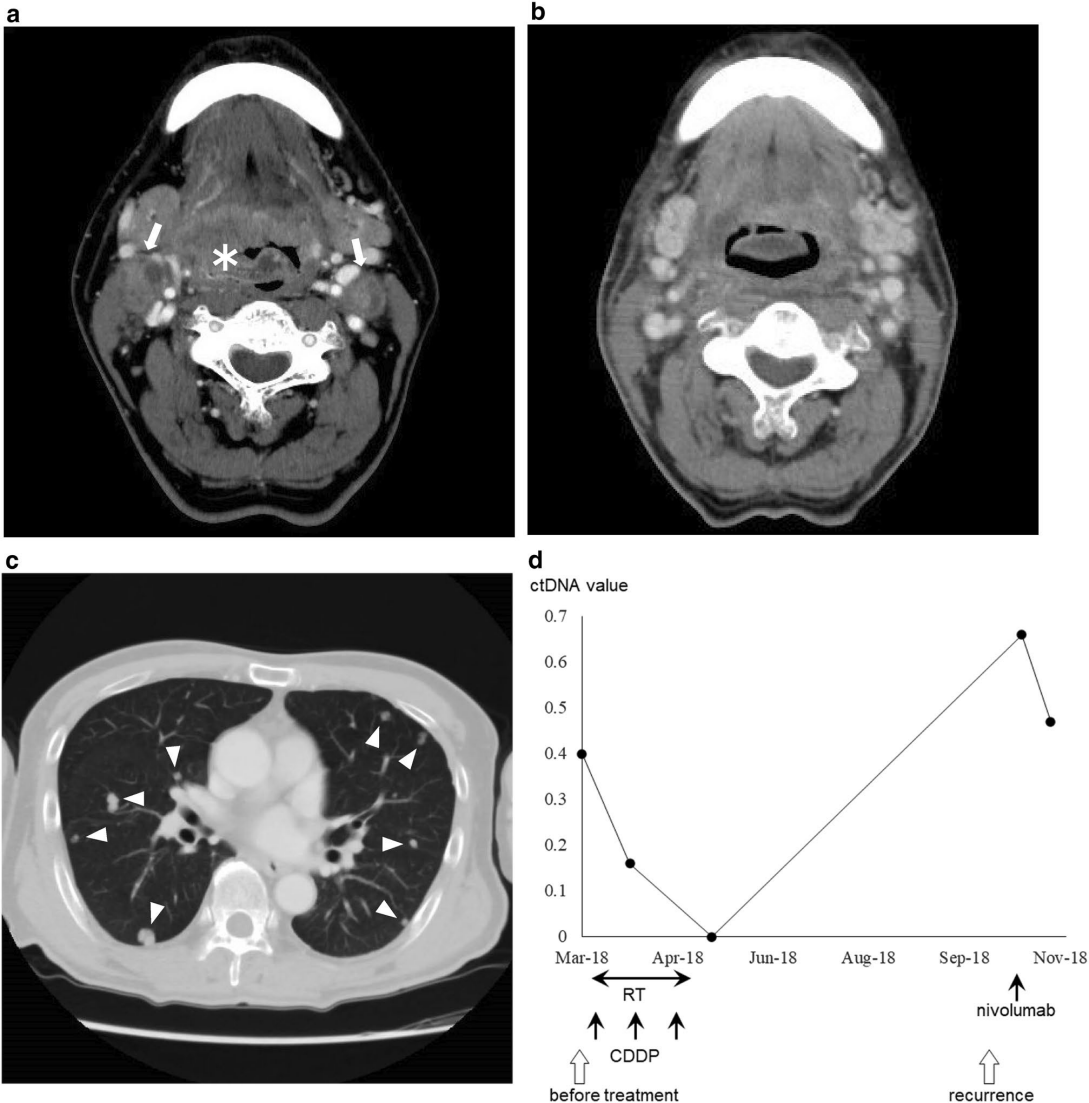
(a) Pretreatment computed tomography (CT) image shows the tumor (asterisk) extending from the right lateral wall to the anterior wall of the oropharynx and cervical node metastasis (arrows). (b) CT image after initial treatment shows complete response. (c) CT image shows multiple lung metastases (triangles). (d) The pretreatment circulating tumor DNA (ctDNA) was positive and turned negative after initial treatment with concomitant chemoradiotherapy with CDDP; ctDNA was positive again at the time of local and distant recurrence and remained positive despite the administration of immune checkpoint inhibitor therapy with nivolumab.
